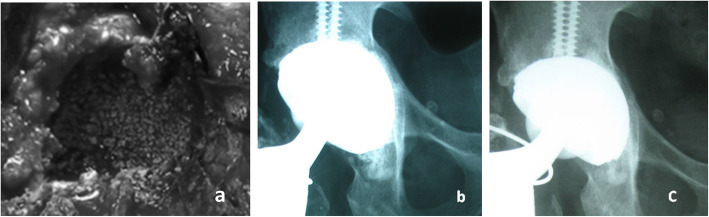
a Intraoperative view of acetabular reconstruction with bone graft substitute in 44 year-old patient. b Postoperative radiograph presents well-fixed socket with impacted bone graft substitute. c After 10 years acetabular cup is still well-fixed with graft bonded with host bone
